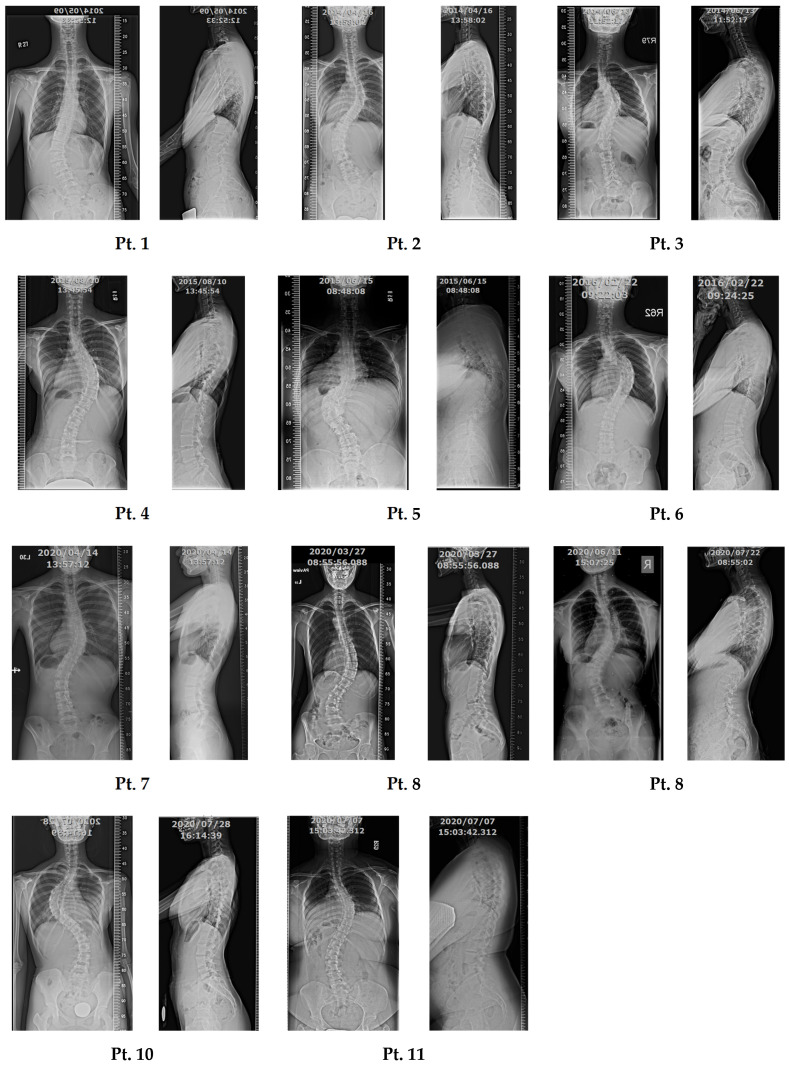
X-rays of 11 AIS patients. Front- and side-view X-rays of each patient were recorded before surgery. The X-ray is used to determine the severity of scoliosis.
